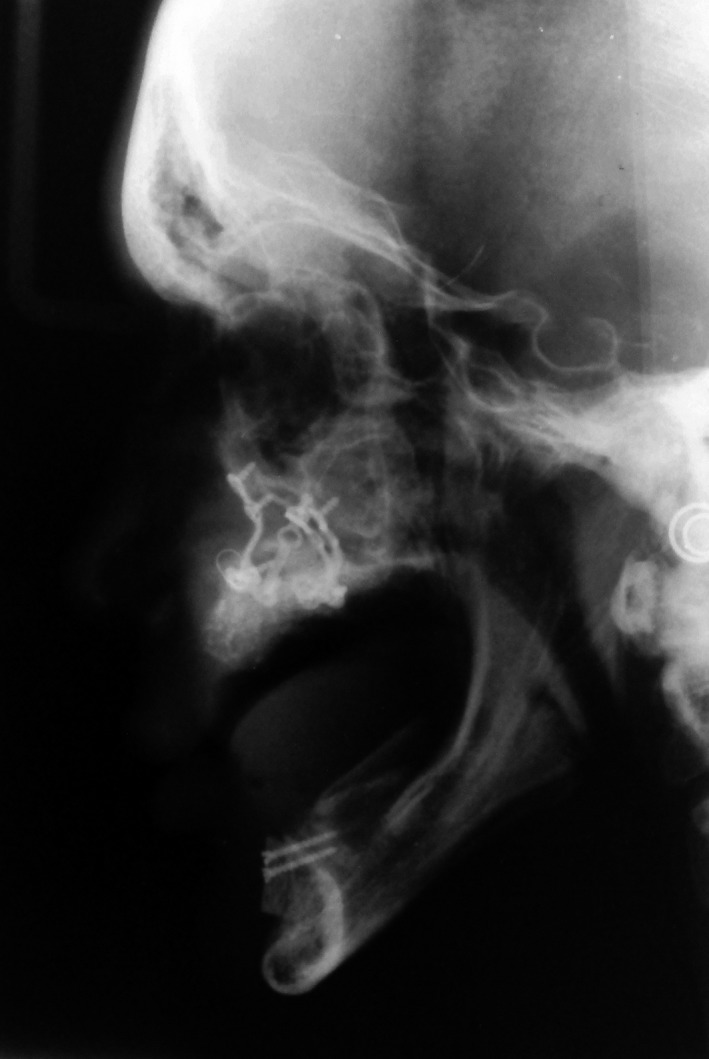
Pretreatment radiographs. Maxillary advancement surgery and augmenting iliac crest bone grafts of both arches.
